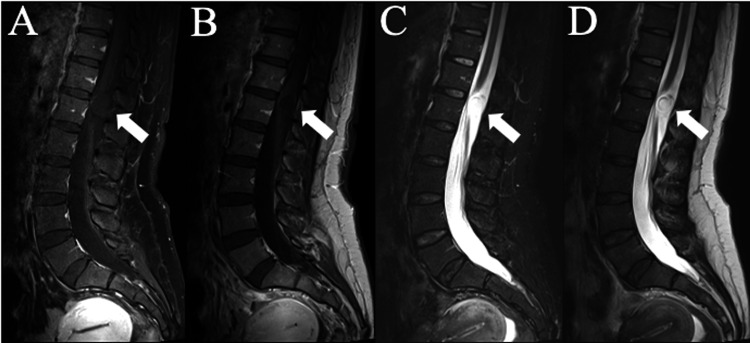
Sagittal thoracic and lumbar MRIs demonstrating T12-L1 intradural extramedullary lesion The sagittal thoracic and lumbar spine MRIs demonstrate the T12-L1 lesion (white arrow) found on imaging. (A) is a sagittal T1 MRI of the thoracic and lumbar spine with gadolinium. (B) is a sagittal T1 MRI of the thoracic and lumbar spine without gadolinium. (C) is a sagittal T2 STIR MRI of the thoracic and lumbar spine. (D) is a sagittal T2 MRI of the thoracic and lumbar spine.
